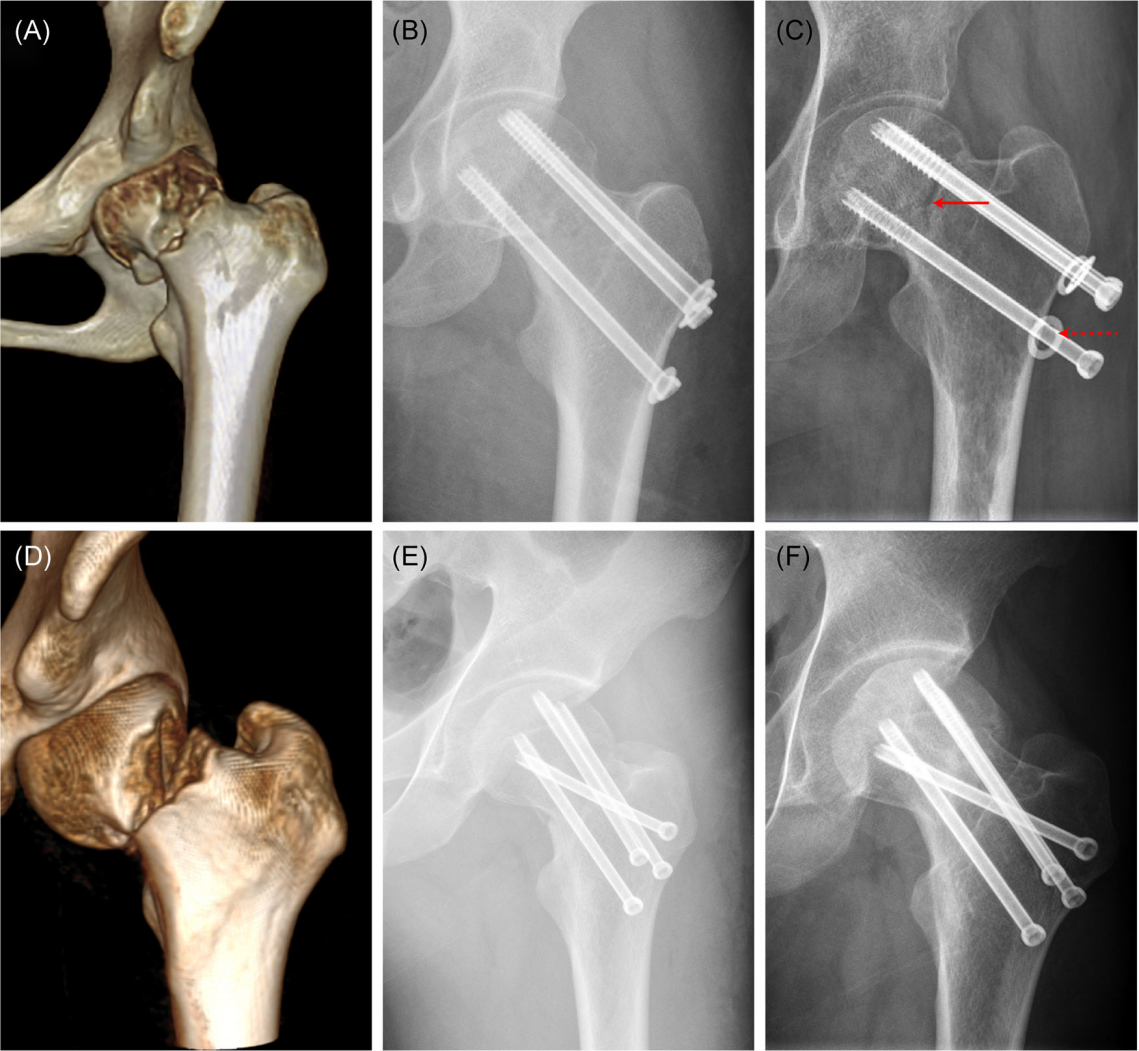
Clinical observation of alpha and invert triangle fixation strategies. (A) Preoperative computed topography of a 34‐year‐old female with a Pauwels type‐3 femoral neck fracture. (B) Radiograph on the second day after this patient was treated with traditional three cannulated screw. (C) Postoperative radiograph approximately 14 months after fixation showing non‐union (solid arrow) and screw withdraw (dotted arrow). (D) Preoperative computed topography of another 26‐year‐old male with a vertical femoral neck fracture. (E) Radiograph on the second day after this patient was treated with cannulated screws with an alpha configuration. (F) Radiograph showed fracture union at 13 months postoperatively [Color figure can be viewed at wileyonlinelibrary.com]
